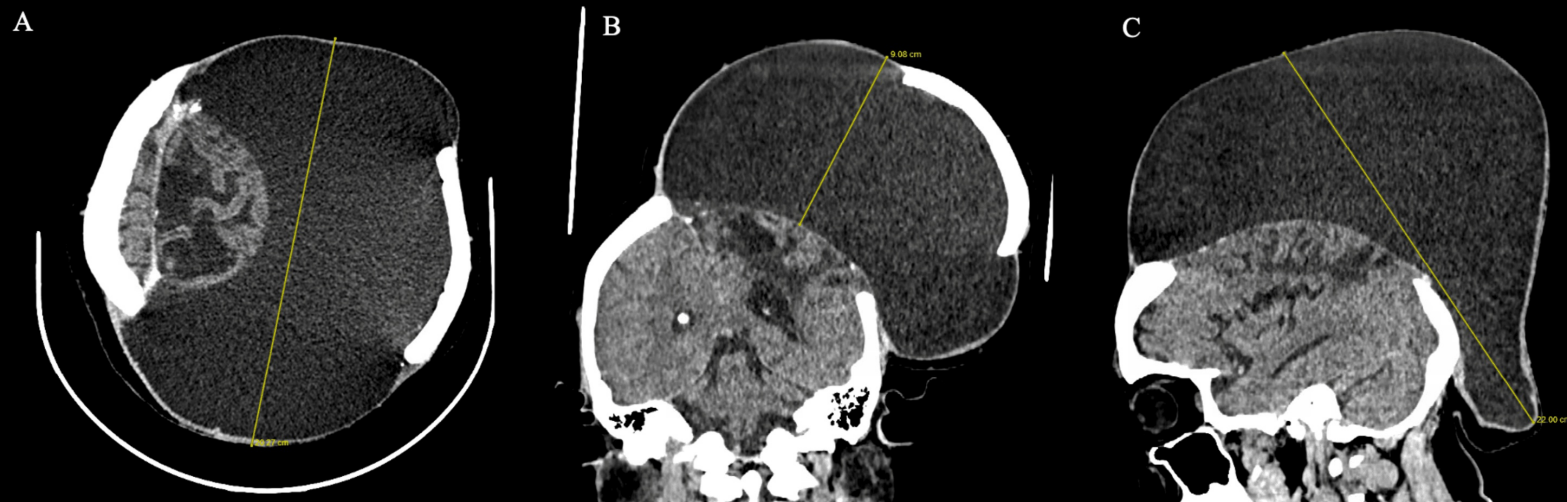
Head CT showing a large well-defined hypodense swelling arising from the craniotomy site (A) Axial section. (B) Coronal section. (C) Sagittal section. The swelling measures approximately 22 x 20 x 9 cm and is elevating the bone flap. Surgical site gliosis is noted.
